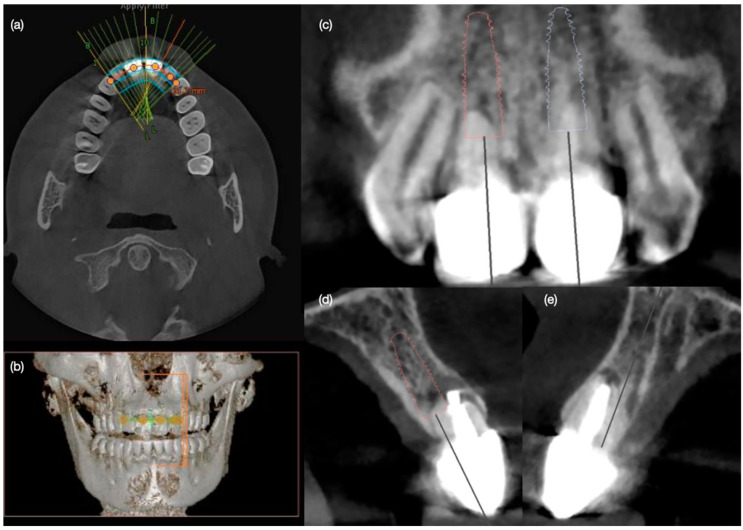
Radiographic evaluation of the central incisors with infection at the apical area. (a) CBCT occlusal view, and (b) frontal view; (c) frontal view and (d) and (e) lateral views of central incisors.
